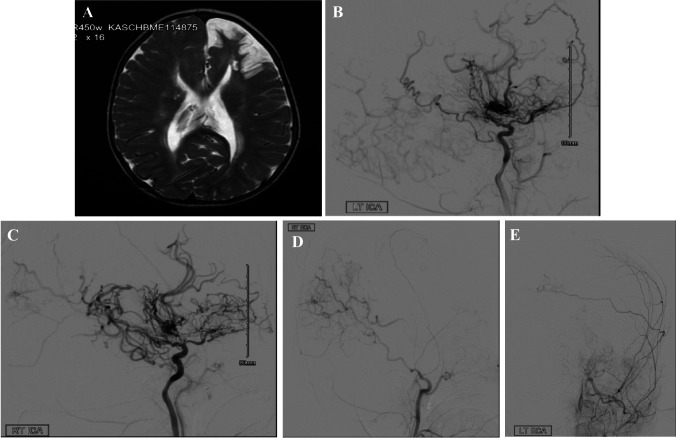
A T2 MRI imaging showing left frontal lobe ischemic insult and atrophy. B Cerebral angiography of left ICA showing stenosis of ICA and collateral vessel formation. C Cerebral angiography of right ICA showing marked collateral vessels of leptomeningeal and lenticulostriate source. D Right ECA (occipital artery) vascularization through the shunt burr hole. E Left ECA (occipital artery), crossing to the contralateral side to vascularize through the shunt burr hole
